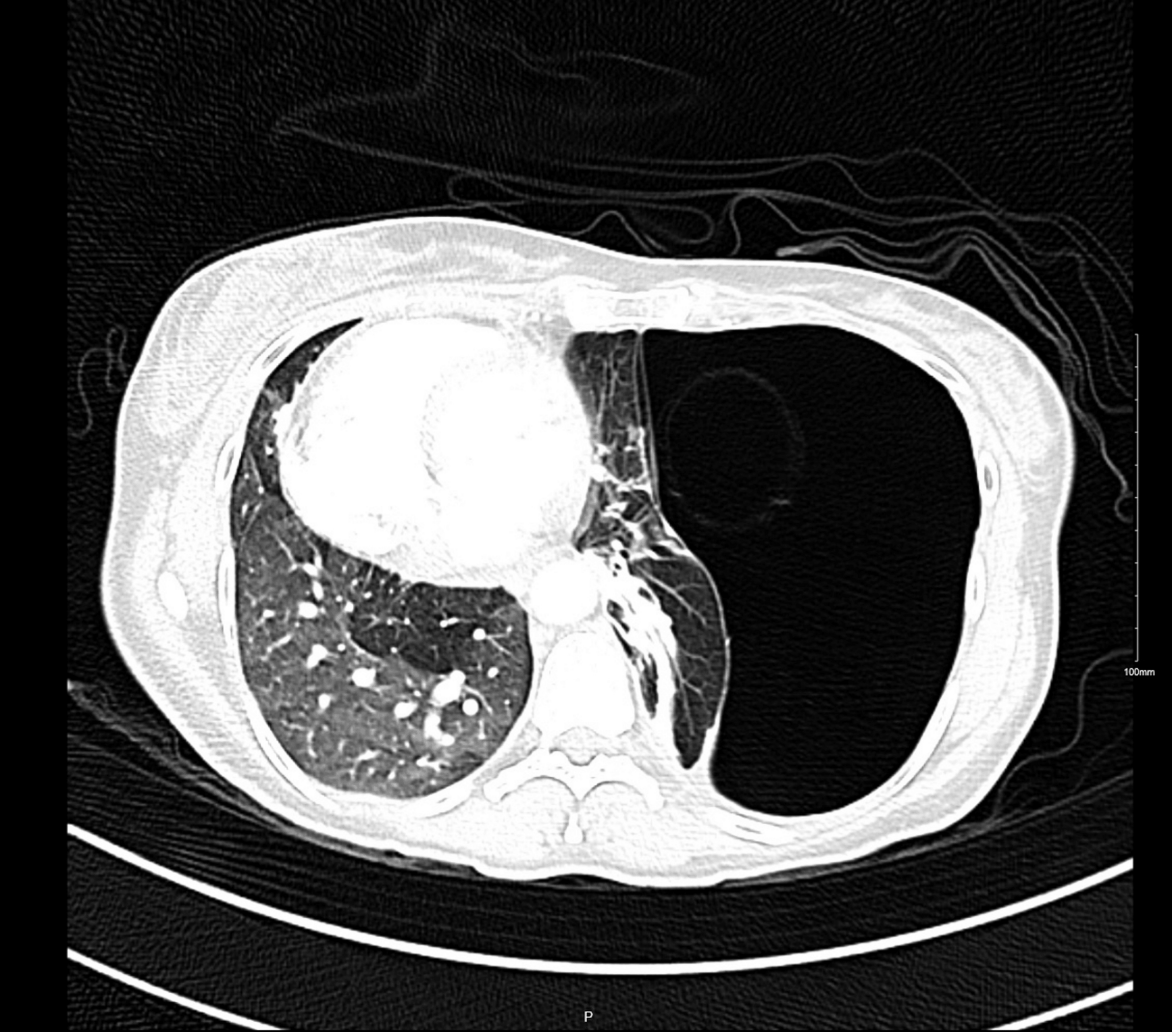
Chest CT scan revealing large bullae on the surface of the left lung, with marked mediastinal shift toward the contralateral side
